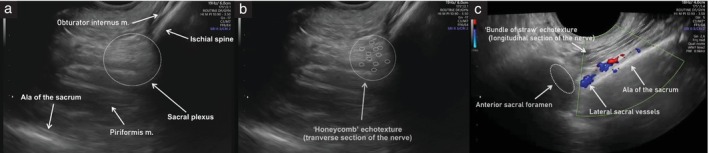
Ultrasound images of left sacral plexus. (a) Anatomic structures of the greater sciatic foramen with sacral plexus (dotted circle). (b) Transverse section of the nerves, exhibiting honeycomb‐like echotexture (dotted circles). (c) Longitudinal section of the nerves, exhibiting bundle‐of‐straw echotexture (dotted parallel lines); note, the sacral plexus can be traced up to the anterior sacral foramen (dotted oval) to identify the sacral nerve roots. m., muscle.
